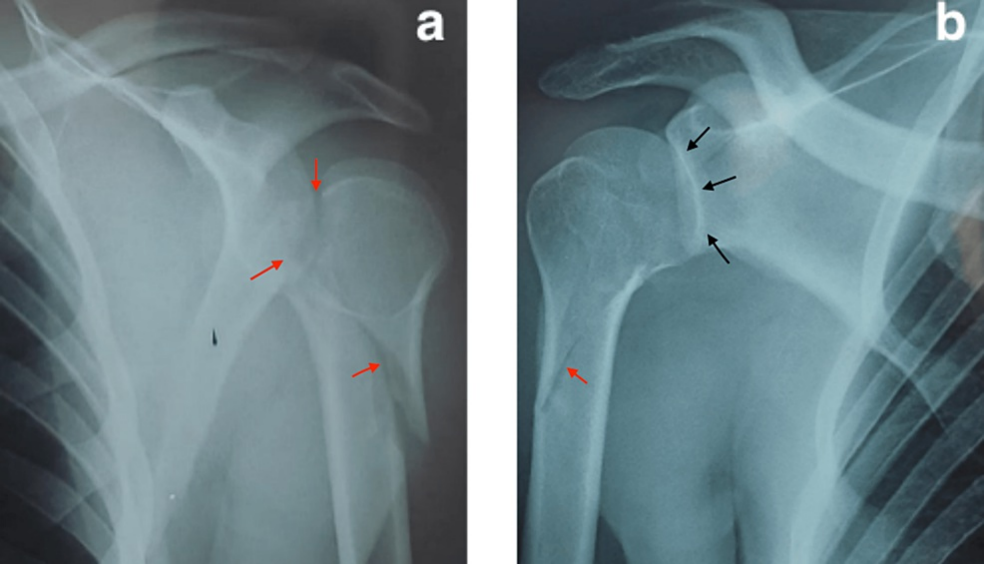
X-rays of the right shoulder after the trauma showing a fracture (red arrow) of the right proximal humerus, with a posterior dislocation (black arrow) of the humeral head.
